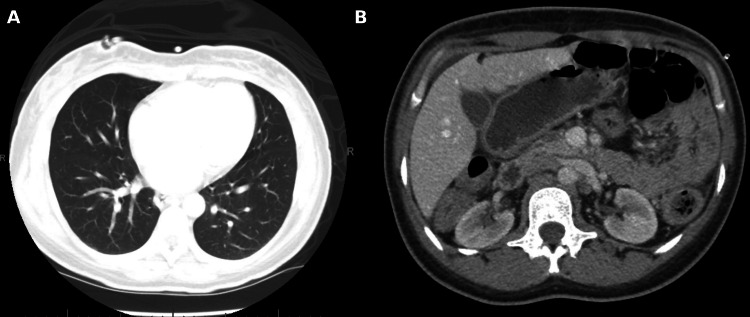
Contrast-enhanced axial CT of the chest (A) and abdomen (B) demonstrating no evidence of pulmonary or hepatic metastatic disease at diagnosis, consistent with clinical stage cT2N1M0.
